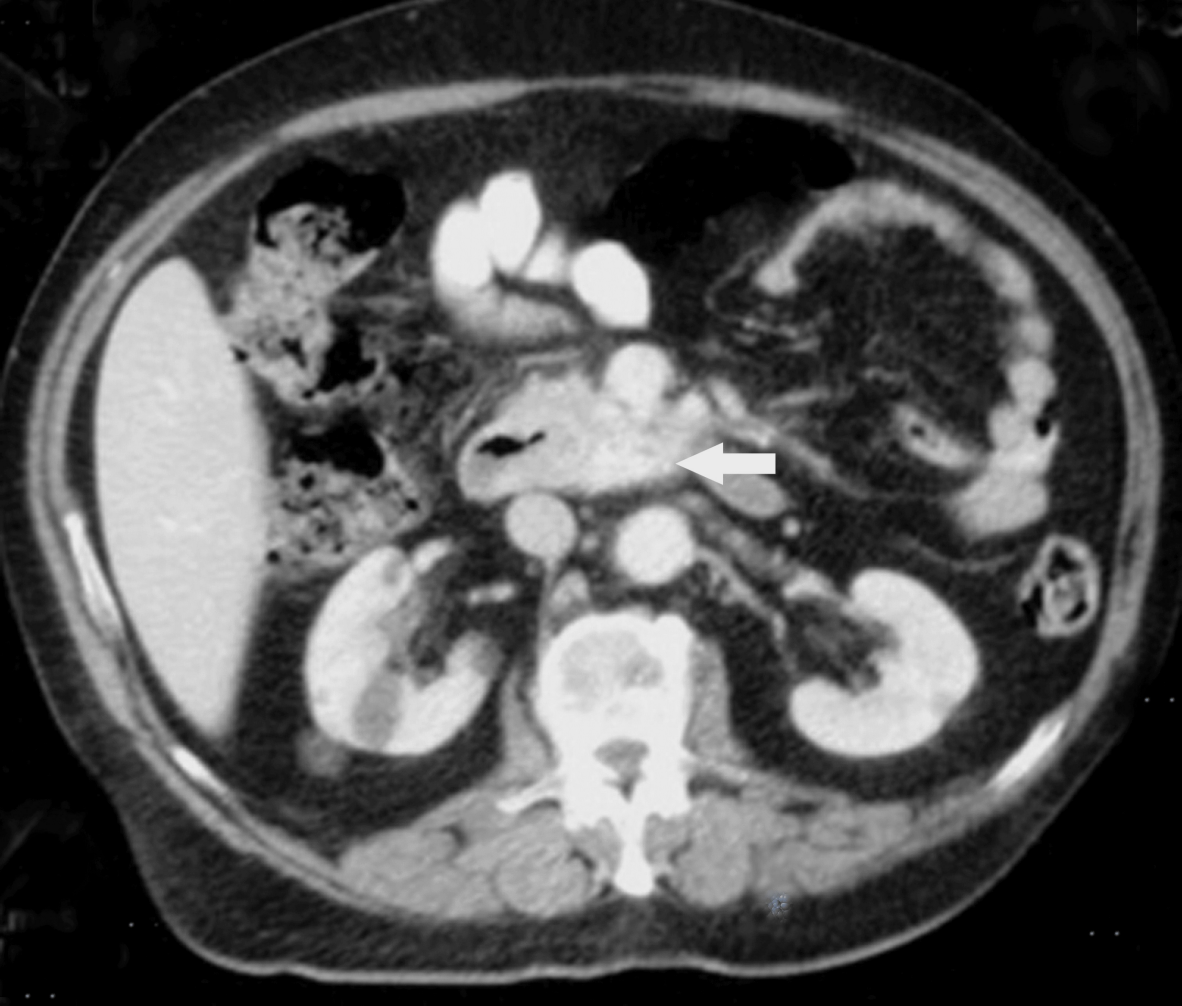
Contrast-enhanced computed tomography of the abdomen. Showing increased vascularity in the pancreatic head and the uncinate process (arrow).
